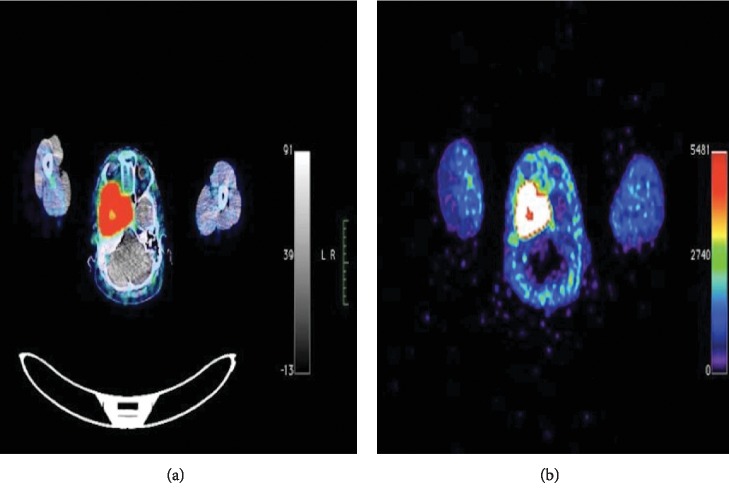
Transversal image from the full body PET-CT scan with 68GA-DOTATATE (a) and 131-MIBG SPECT (b) showing intense uptake in the skull base tumor.
